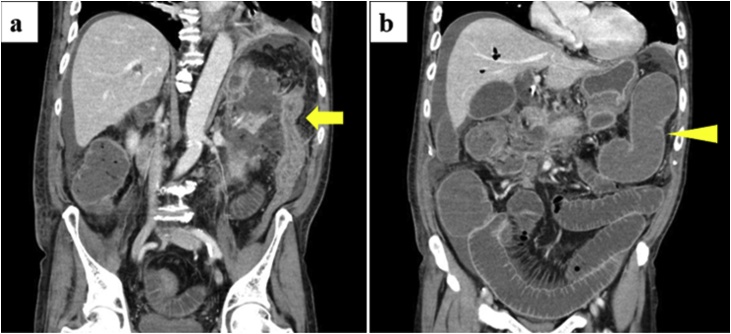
Contrast enhanced abdominal computed tomography scan revealed a stenosis in the descending colon near the splenic flexure region (arrow); b proximal dilated colon (arrowhead) and small bowel.
